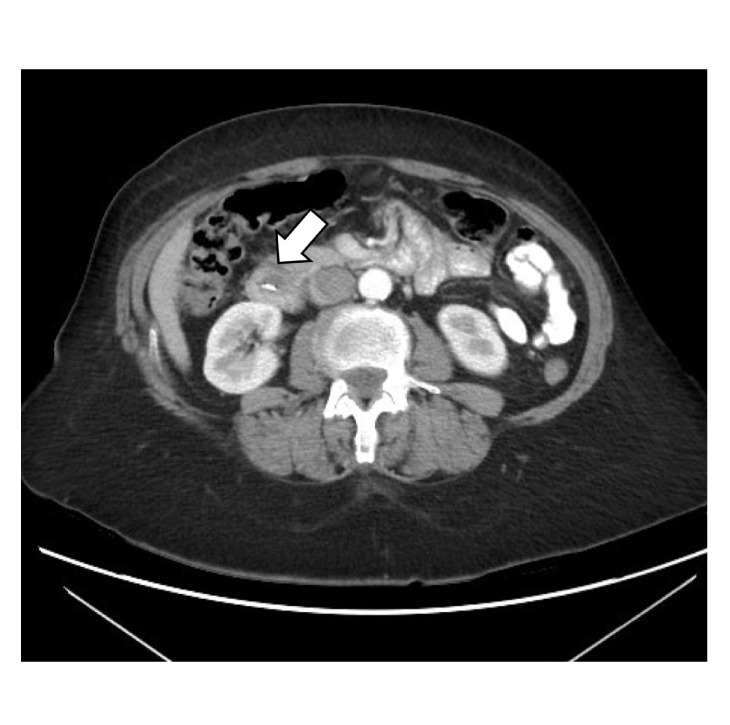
Abdominal CT showing a radiodense foreign body by the ampulla of Vater, most likely a migrated surgical clip from the prior cholecystectomy (arrow).
